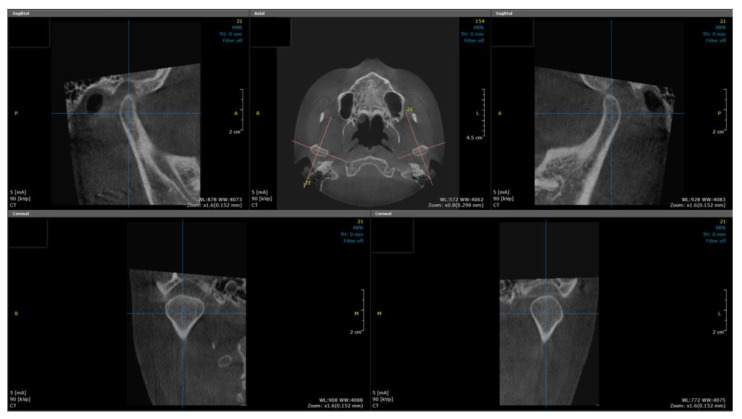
Sagittal and coronal reconstruction of a cone beam computed tomography.
